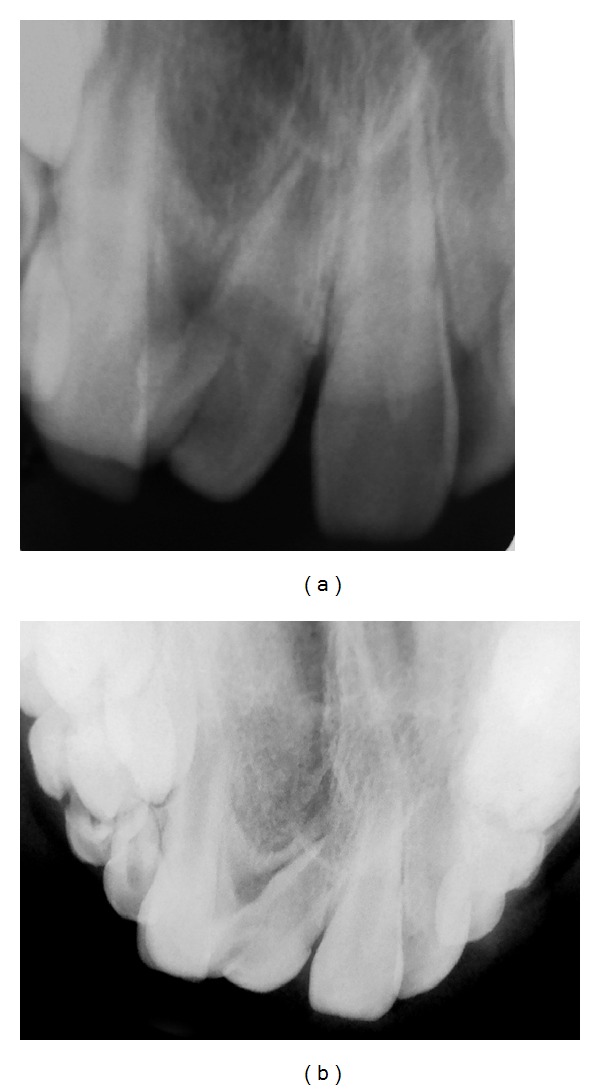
Radiographic appearance of fused tooth.
